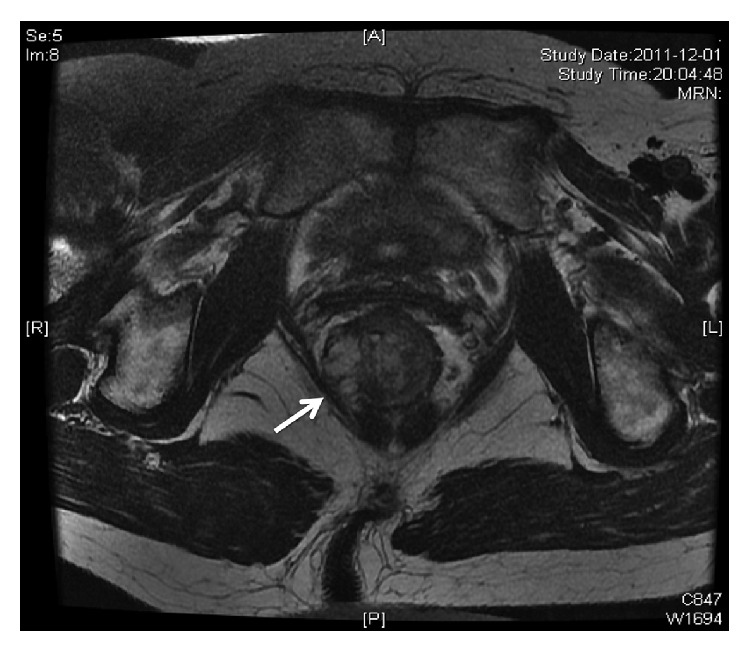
T2-weighted axial thin section magnetic resonance imaging scan. This scan shows a low rectal tumor with involvement of the potential circumferential resection margin (white arrow).
